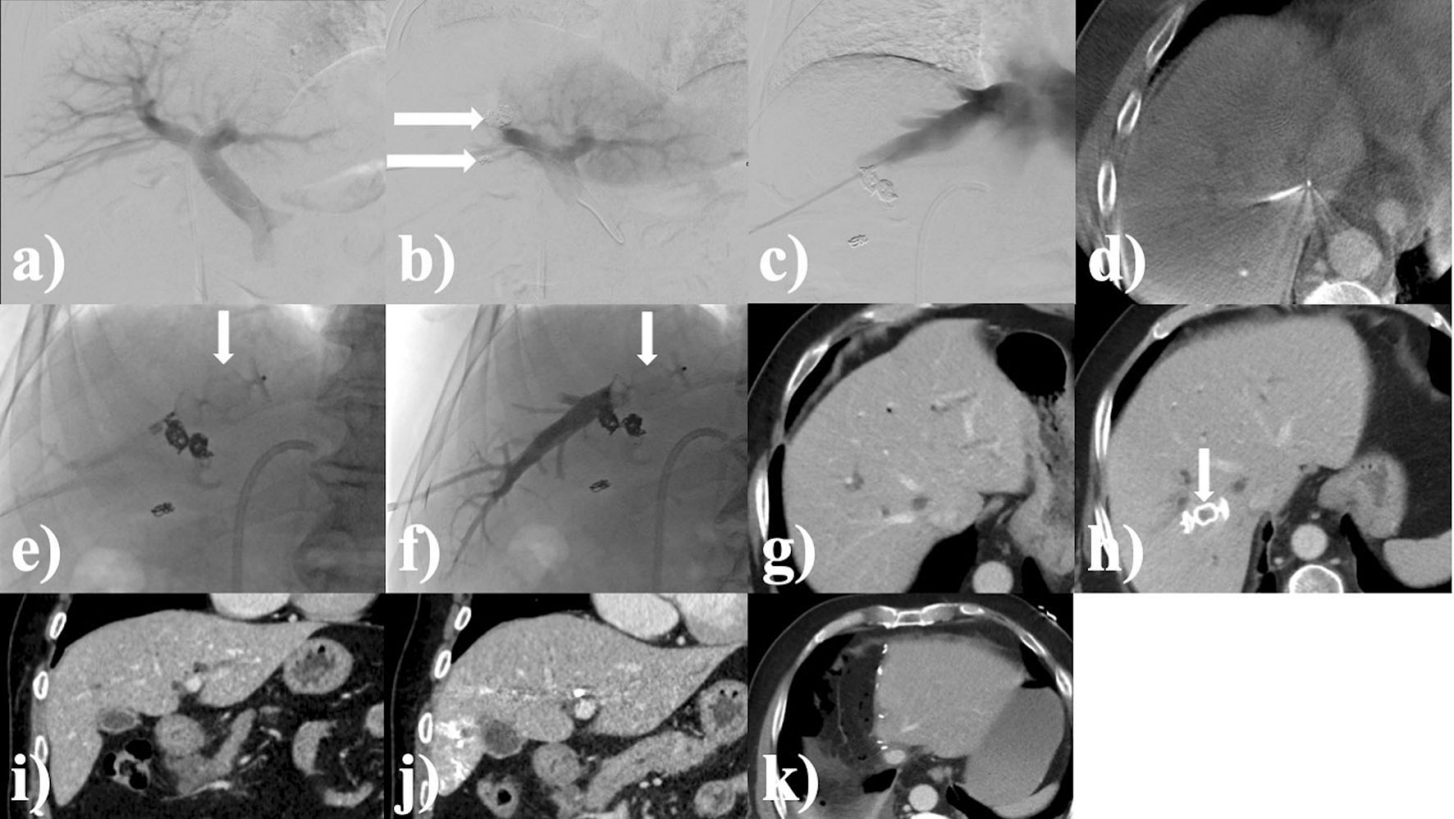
Example case. A 75-year-old man with cholangiocarcinoma (CC, Klatskin tumor type IIIa). Total radiation dose applied during intervention: 64.6 Gy*cm^2^, fluoroscopic time of intervention: 17 min; hypertrophy time: 28 days; relative hypertrophy rate of liver segments II + III: 68.2%, relative hypertrophy rate of liver segments II–IV: 68.1%; LiMAx score before surgery: 291 µg/h/kg. a Digital subtraction angiography (DSA) of the portal vein before embolization (PVE) after ultrasound-guided, transhepatic puncture. b DSA portography after embolization of the right portal vein branch with coils and particles. c DSA venography of the right hepatic vein after ultrasound-guided transhepatic puncture. d Verification of catheter position in the right hepatic vein by cone-beam CT. e Fluoroscopic positioning of the plug in the central right hepatic vein. f DSA of embolization of the puncture tract including peripheral vascular segments with a mixture (ratio of 2:1) of *n*-butyl-2-cyanoacrylate and ethiodized oil. g Planning CT before LVD in venous contrast phase. h CT in venous phase after hypertrophy time with verification of correct plug positioning in the right hepatic vein. i Planning CT before intervention in coronal orientation. j CT after hypertrophy time in coronal orientation with subjective hypertrophy of the left liver lobe. FLR hypertrophy (segments II + III) in this case was 68%. k CT after extended right hepatectomy horizontal arrows: coils, vertical arrows: plug
